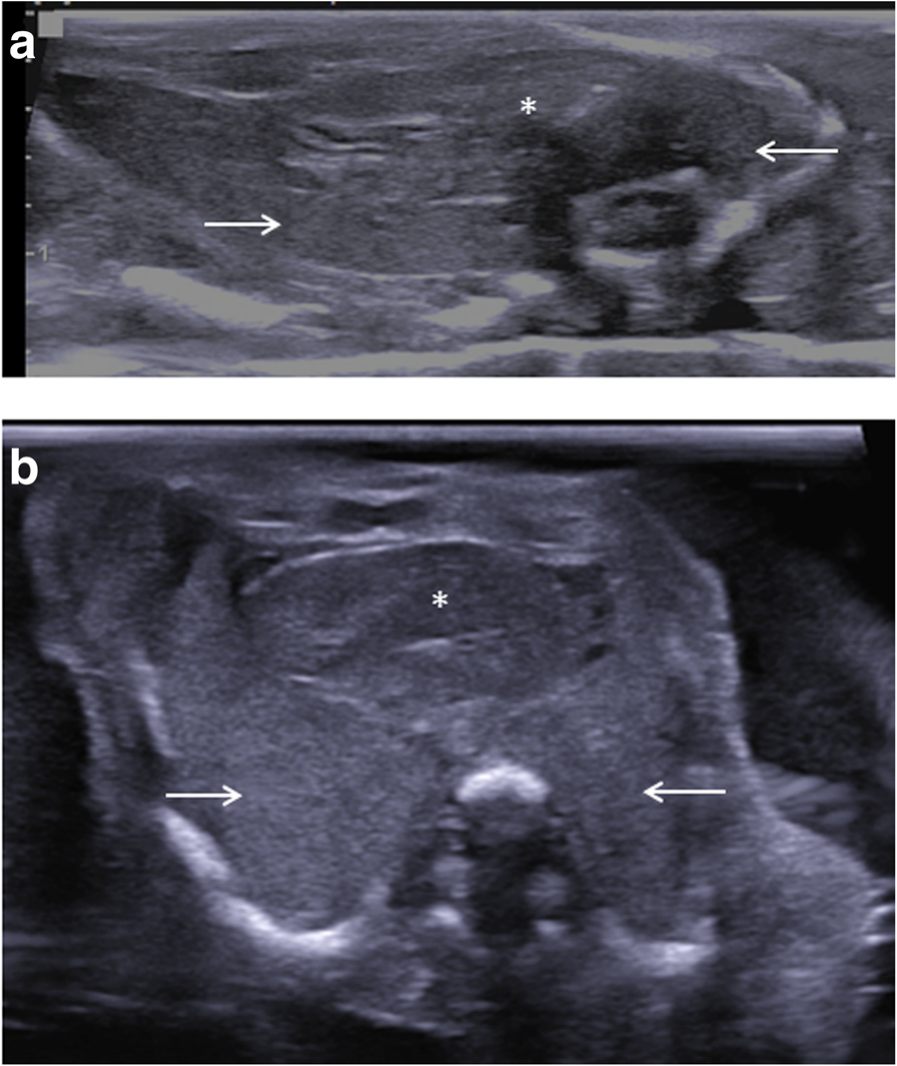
Two transverse postmortem ultrasound images of the chest in the same 23-week gestational-aged foetus, obtained 4 days after death. Postmortem ultrasound performed outside the water bath (a) and with foetus in the water bath (b). Notice how the thoracic cage was collapsed both due to body laxity and transducer compression in image (a), but how there is improved visualisation of the heart (asterisk) and lungs (white arrows) using the water bath (b) as the chest expands
